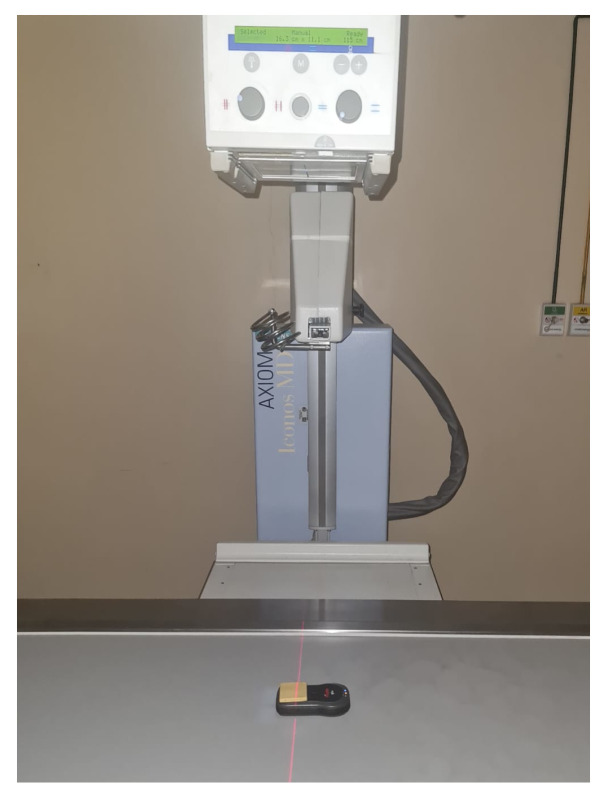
X-ray exposure depicting the evaluation of shielding effectiveness using a 4 mm sample placed on the X-ray QA meter.
